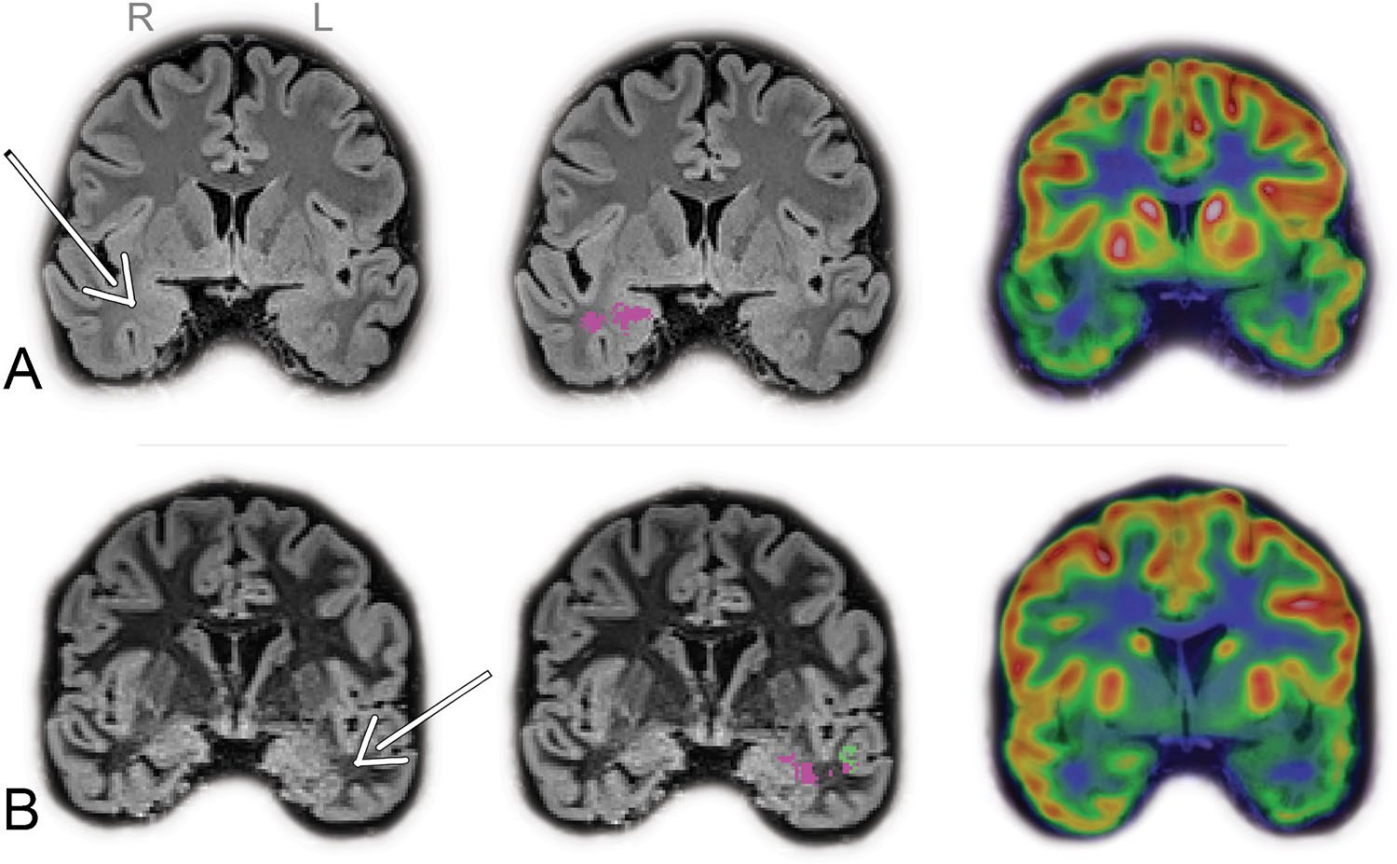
Examples of additional lesions identified on second look MRI, showcasing FLAIR/DIR images on the left side, FDG-PET on the right side, and their overlaid abnormalities detected by the toolboxes in the middle: MELD (green), and MAPcombined (purple). (A) A patient with right TLE in which only right hippocampal sclerosis was initially detected during the clinical work-up. MELD and MAPcombined revealed abnormal clusters in the right temporal pole. (MELD cluster located more anteriorly to the coronal slice not shown). The second look MRI confirmed the presence of a subtle blurring between the grey and white matter in the right temporal pole (arrow), a finding concordant with the hypometabolism observed in FDG-PET imaging in the same area of the right temporal pole. (B) A patient with left TLE where initial MRI findings were limited to left hippocampal sclerosis. Abnormal clusters were identified in the left amygdala (MAPcombined) and white matter of the left temporal pole (MELD and MAPcombined). The second look on MRI confirmed the presence of a subtle increased signal intensity in the white matter of the left temporal pole (arrow), consistent with the hypometabolism seen in the corresponding area in the FDG-PET image
